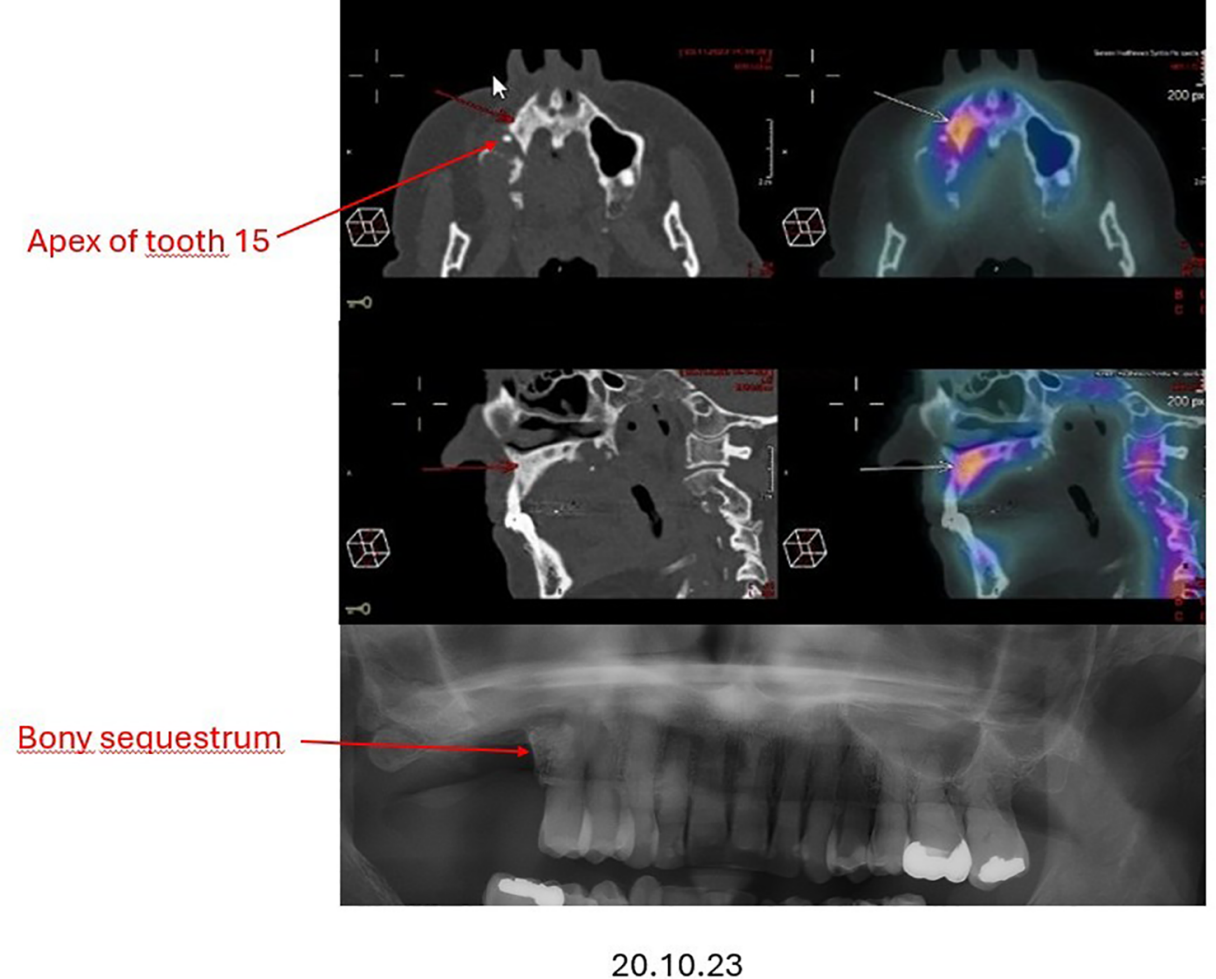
Osteonecrosis of the jaw in PET-CT and OPG.
